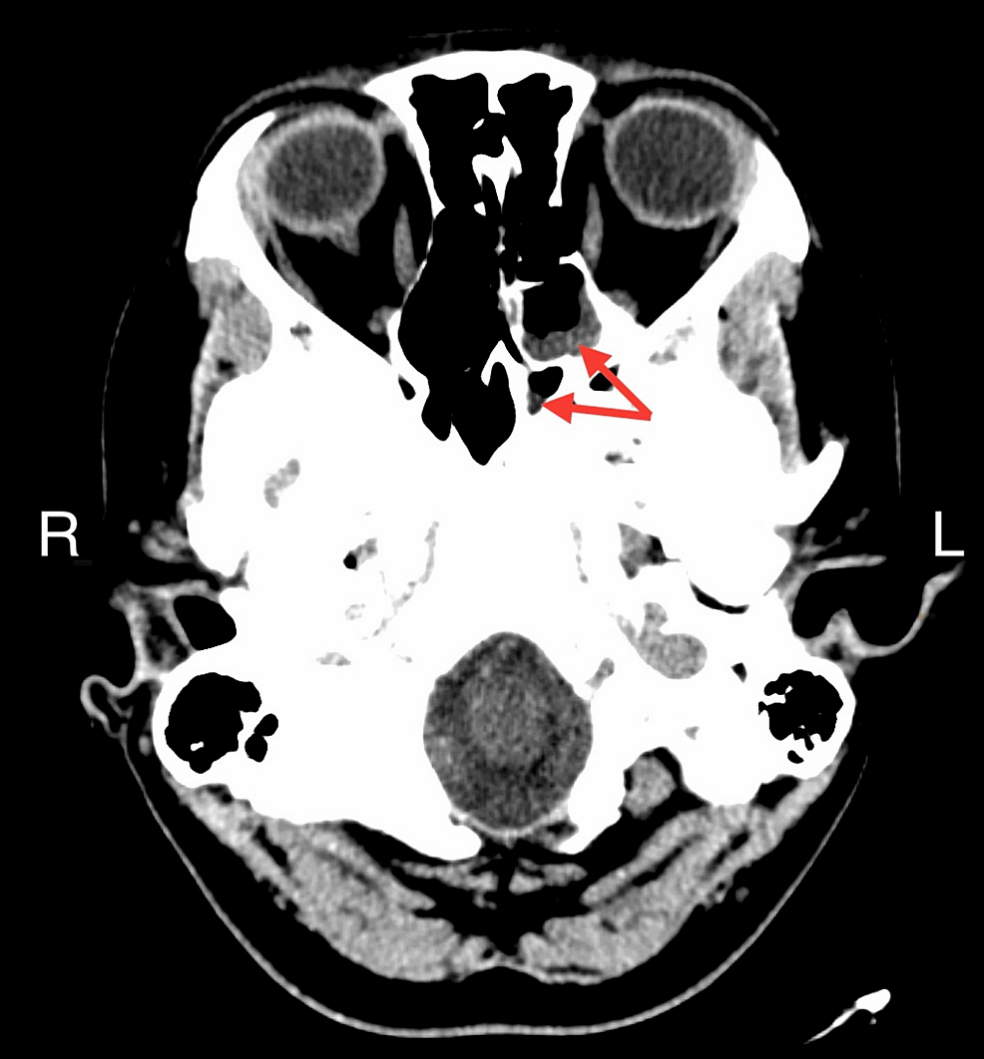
CT scan of the head Red arrows point to the left ethmoid and sphenoid sinus with mucosal thickening suggesting sinusitis.
